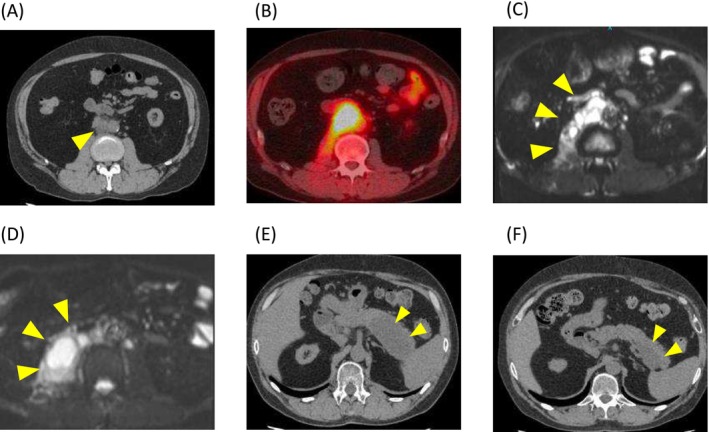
CT and MRI images. (A) At the time of initial presentation, enlarged retroperitoneal lymph nodes were observed. (B) 18F‐fluorodeoxyglucose positron emission tomography image showed increased fluorodeoxyglucose uptake in the retroperitoneal lymph nodes. (C) Post‐hospitalization MRI showed a multifocal abscess. (D) The abscess continued to increase after anti‐tuberculosis drugs were started. (E) Remaining abscess in the pancreatic tail was observed at the end of anti‐tuberculosis drugs. (F) The abscess continues to shrink even after the anti‐tuberculosis drugs have been terminated.
